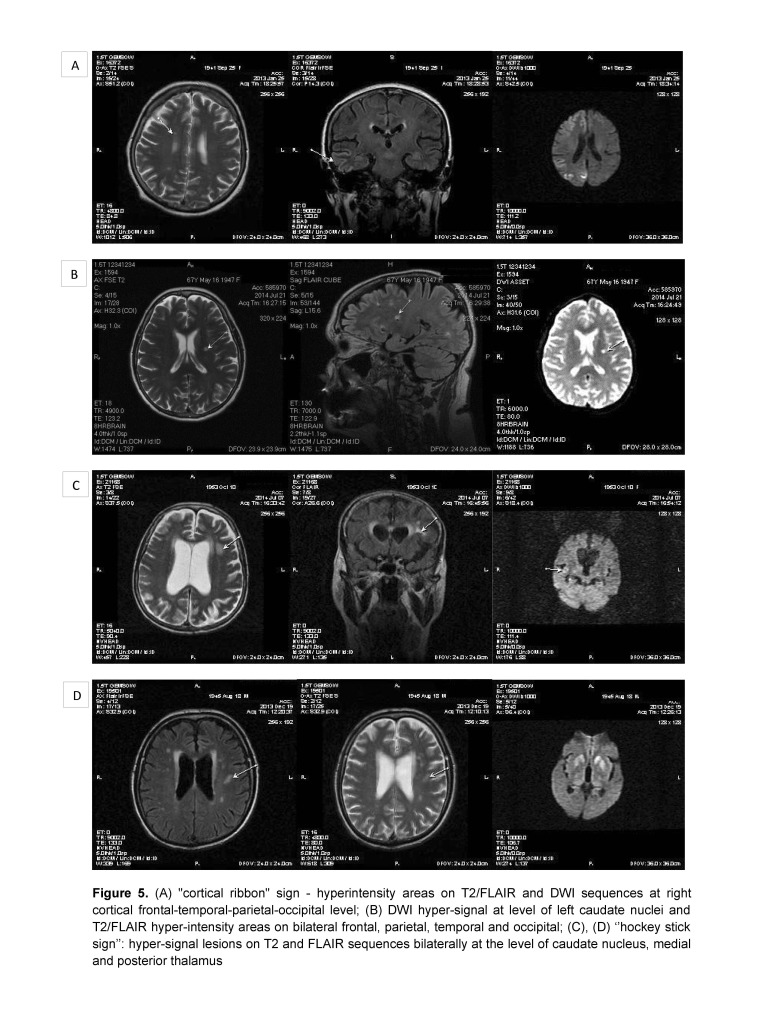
(A) “cortical ribbon” sign – hyperintensity areas on T2/ FLAIR and DWI sequences at right cortical frontal-temporal-parietal-occipital level; (B) DWI hyper-signal at level of left caudate nuclei and T2/ FLAIR hyper-intensity areas on bilateral frontal, parietal, temporal and occipital; (C), (D) “hockey stick sign”: hyper-signal lesions on T2 and FLAIR sequences bilaterally at the level of caudate nucleus, medial and posterior thalamus
